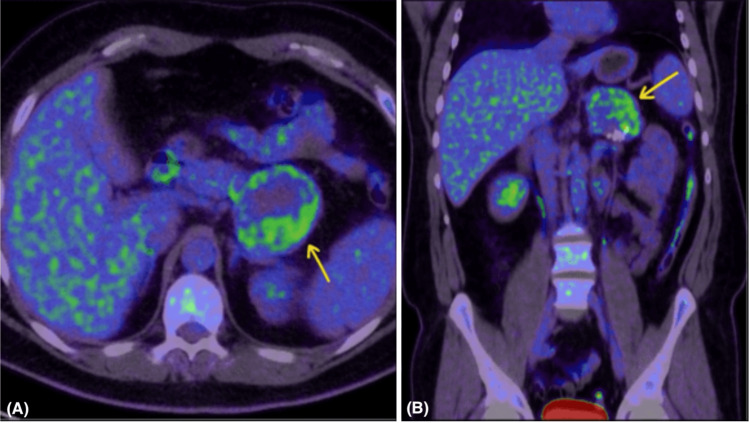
FDG PET/CT images: axial (A) and coronal (B) views of CT soft tissue window showing the pancreatic tail hypodense mass with peripheral and nodular uptake more at the posterior and lateral aspect (yellow arrows). PET/CT: positron emission tomography/computed tomography
